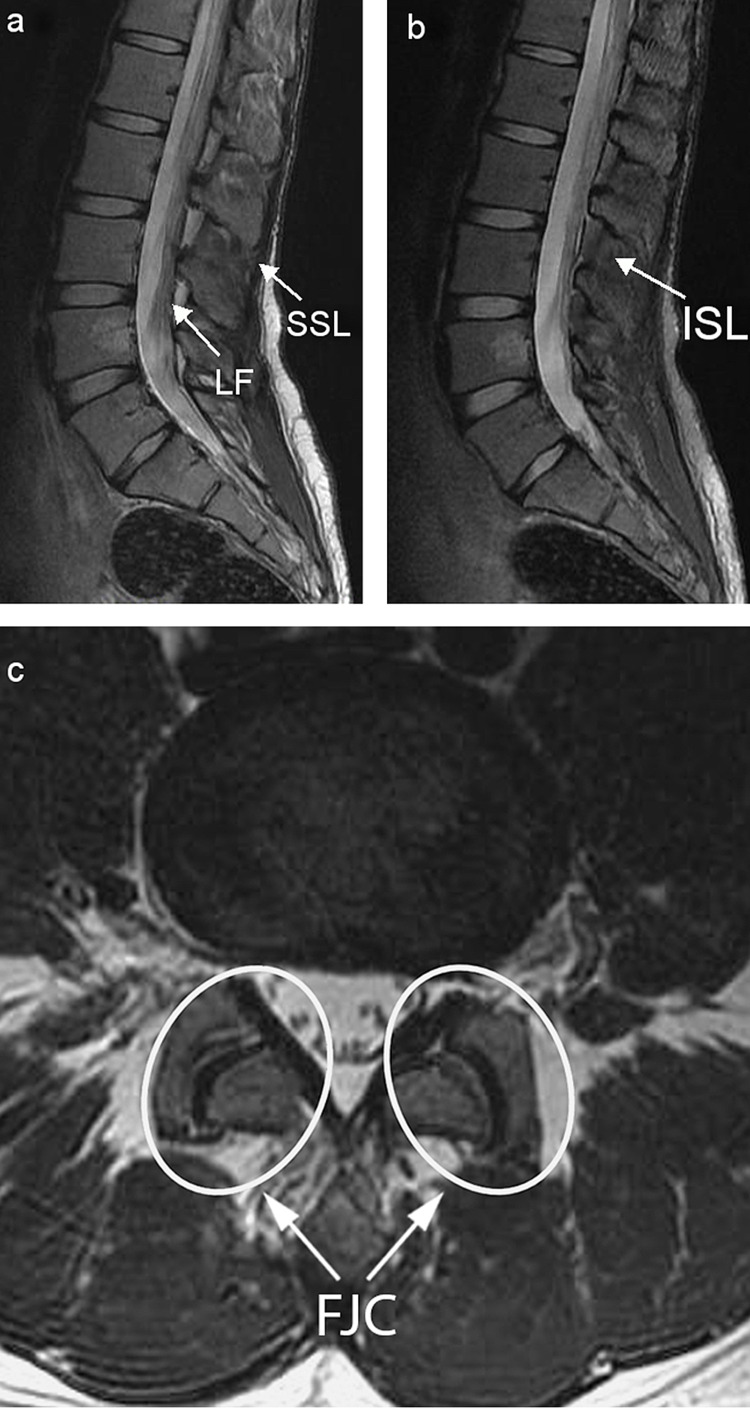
(a) Sagittal T2-weighted lumbar MRI: SSL, LF. (b) Sagittal fat-saturation T2-weighted lumbar MRI: ISL. (c) Axial fat-saturation T2-weighted lumbar MRI: FJC. SSL: supraspinous ligament, LF: ligamentum flavum, ISL: interspinous ligament, FJC: facet joint capsule
